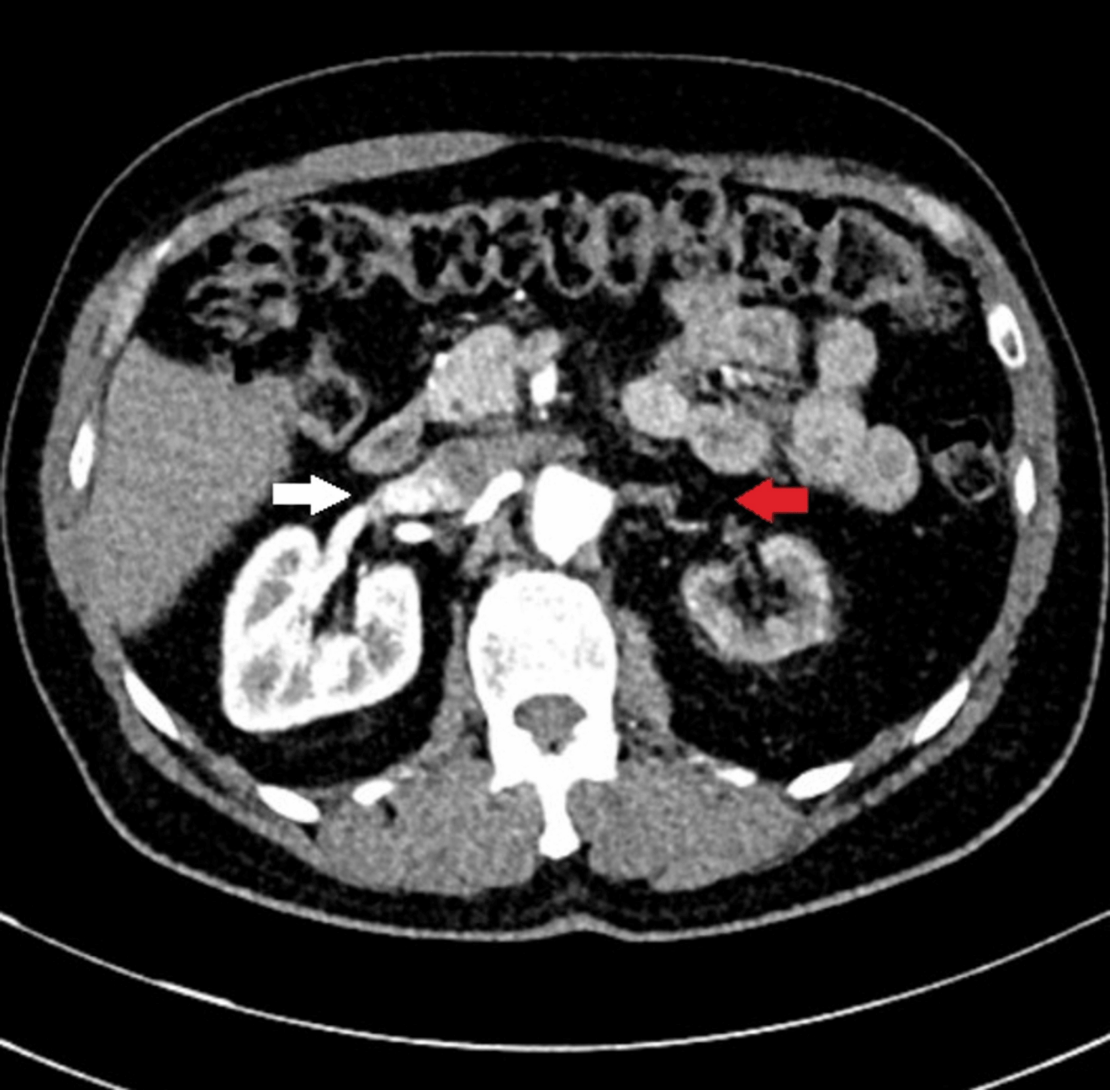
Thoracoabdominal computed tomography angiography showed 40% occlusion of the right renal artery (white arrow) and total occlusion of the left renal artery (red arrow).
